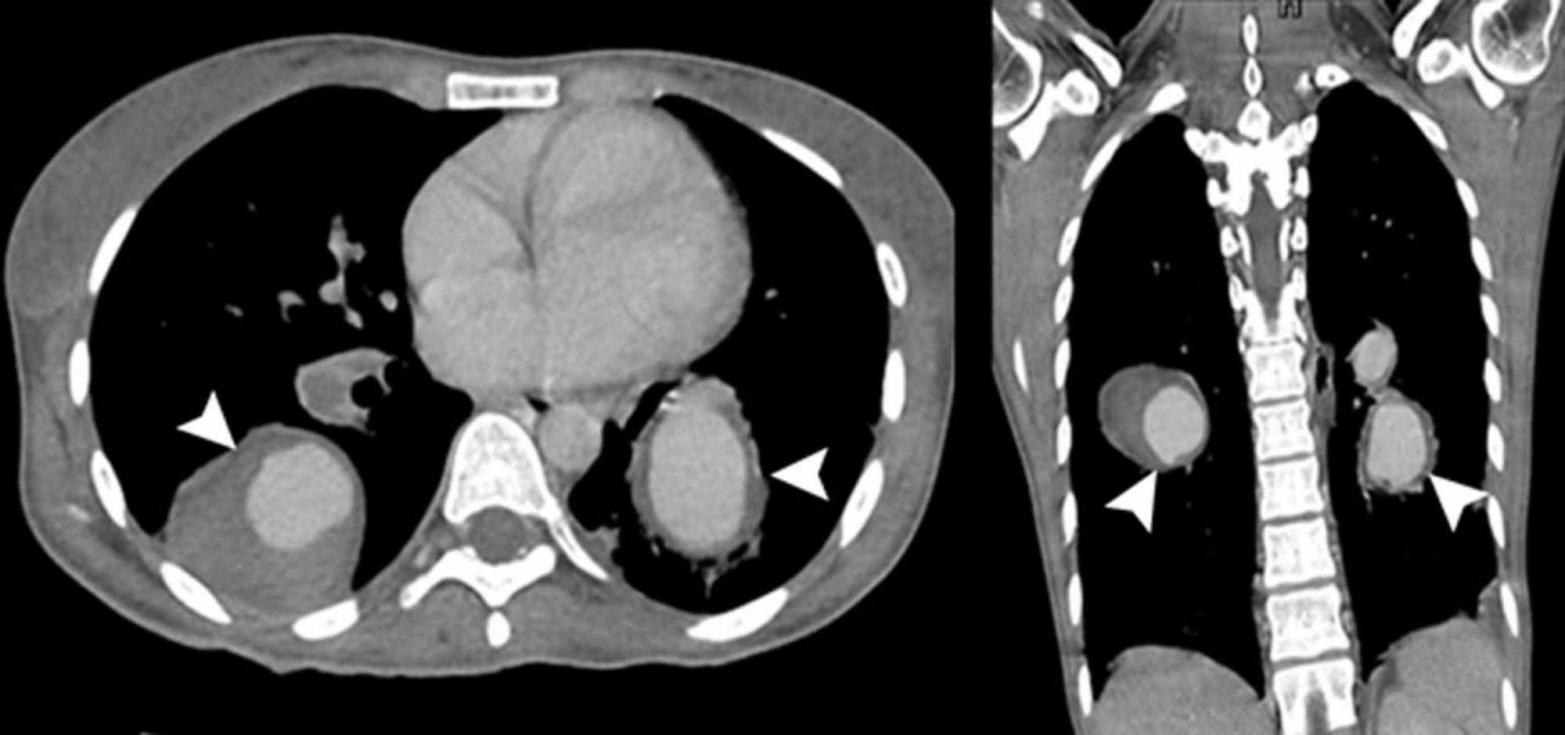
Computed tomography images showing large bilateral partially thrombosed aneurysms (arrowheads) originating from the pulmonary arteries.
